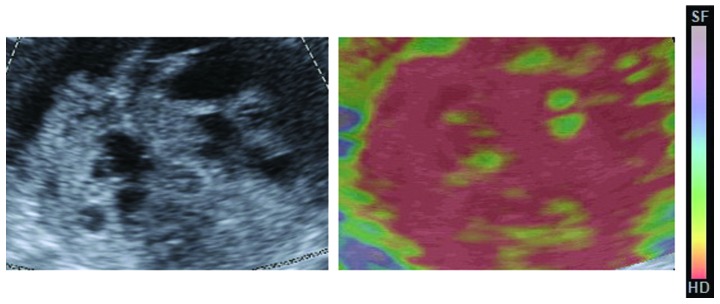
Conventional sonogram and elastogram from the same patient as shown in Fig. 1, but post-NACT, which appears predominantly red and is clearly distinguishable. The case was scored as 4 on the four-point elasticity scale. However, the image from the conventional ultrasound was almost the same as that of the pre-NACT. NACT, neoadjuvant chemotherapy.
